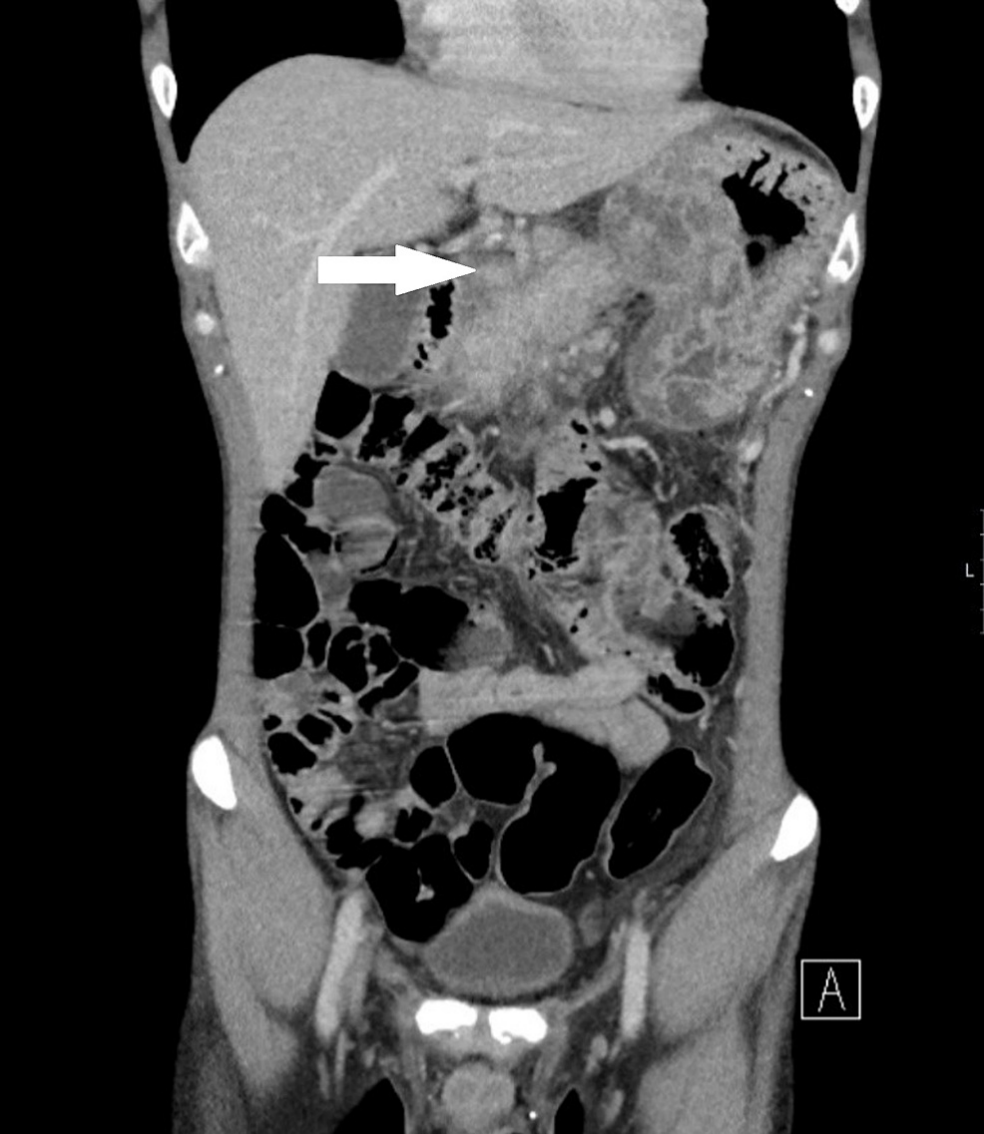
Coronal CT scan showing a large inflammatory peripancreatic fluid collections (white arrow).
